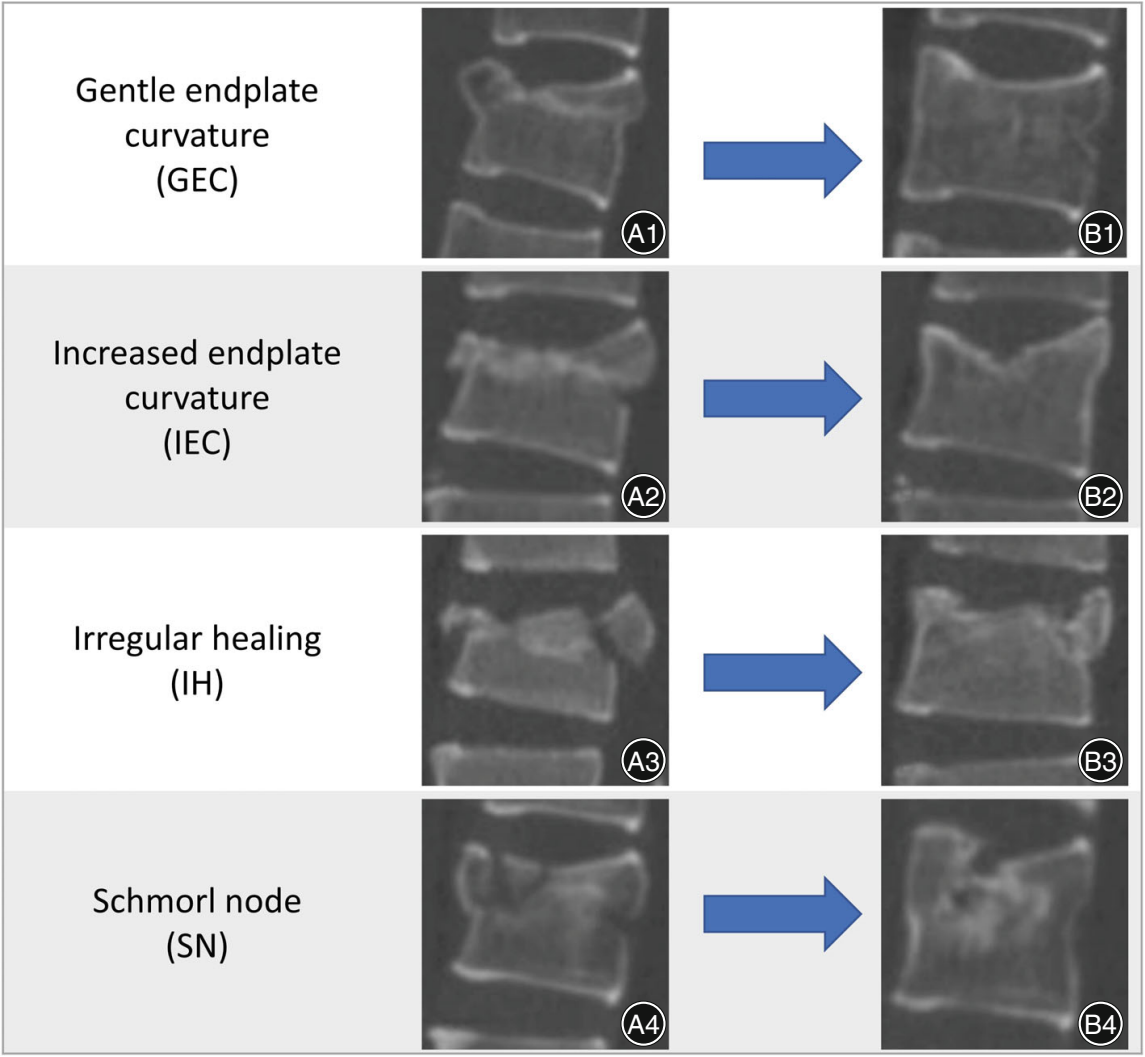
Endplate healing morphology. (A) Preoperative CT 3D lateral view. (B) CT 3D lateral view after the removal of internal fixation. Gentle endplate curvature (GEC): endplate depression repair with a significant increase in depression angle and no significant loss of intervertebral disc (IVD) height. Increased endplate curvature (IEC): endplate depression repair with a significant reduction in depression angle and no significant loss of IVD height. Irregular healing (IH): endplate depression signal irregularity with localized elevation or depression, mild loss of IVD height. Schmorl's node (SN): endplate depression similar to Schmorl's node with a significant loss of IVD height.
